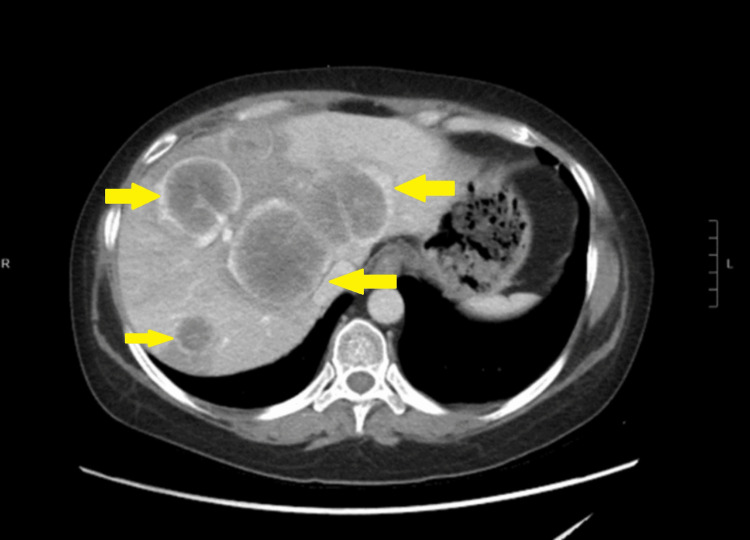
Abdomial CT with contrast done one month prior showed evidence of metastatic disease including multiple large liver lesions.
